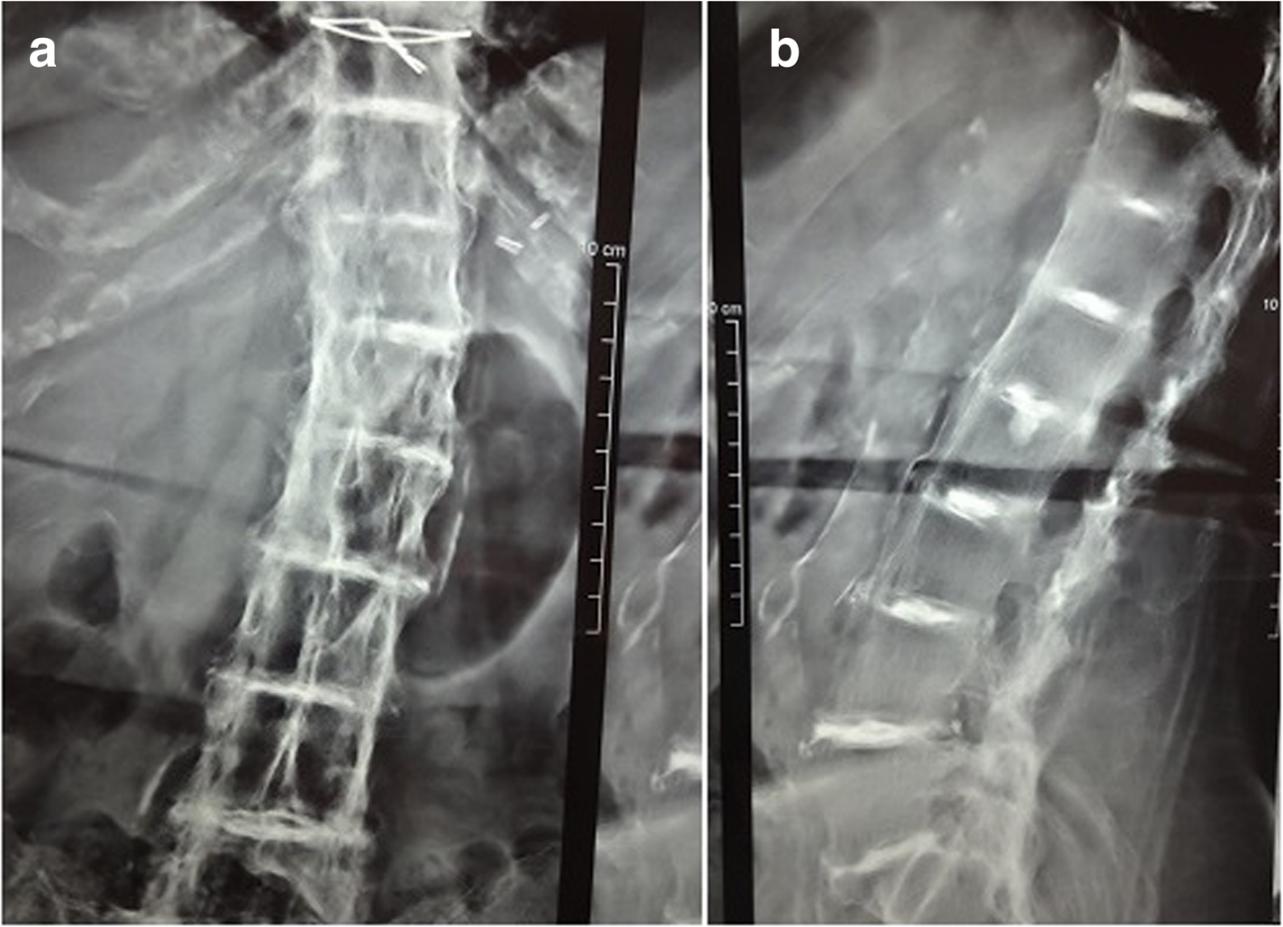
a & b Anteroposterior and lateral spine X-ray films revealed osteoporosis, flattened, and intra-discal calcification (doubling signs), and variable degrees of fusion of the vertebral bodies
